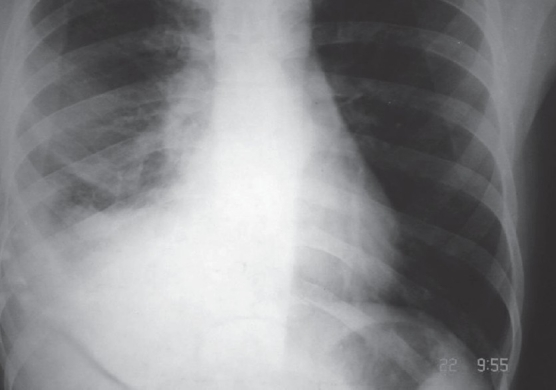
X-ray chest showing right-sided effusion and pneumonitis
